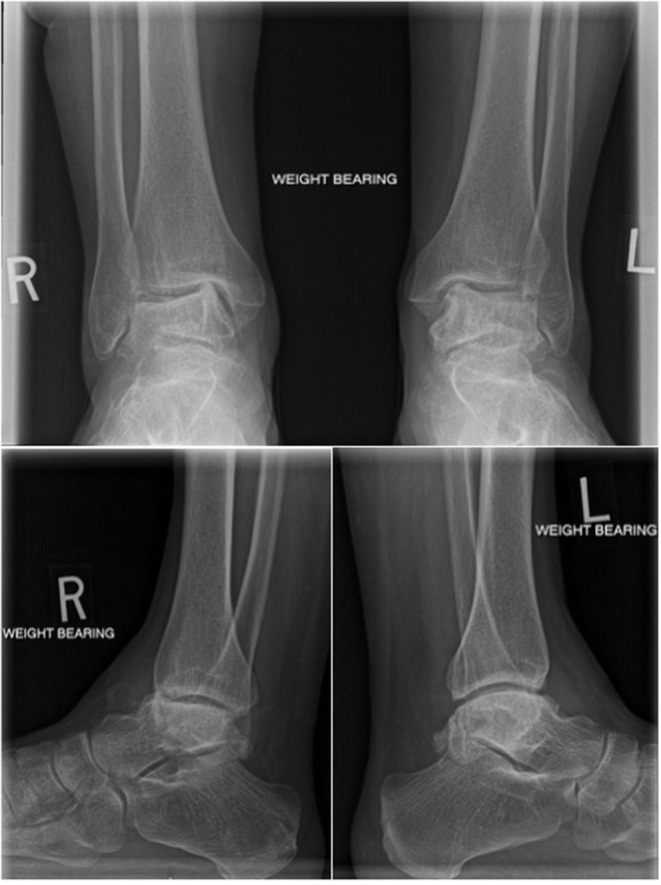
Radiographs at the time of presentation including bilateral AP weightbearing, right ankle weightbearing lateral and left ankle weightbearing lateral views.
